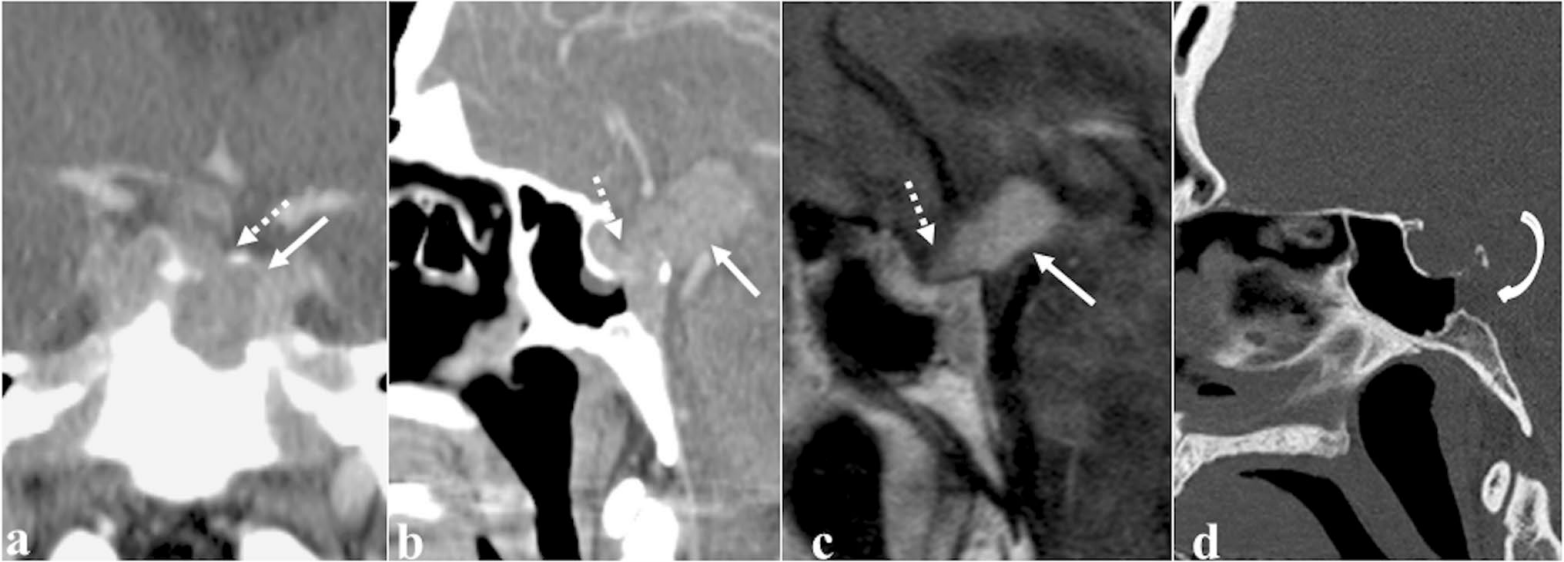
Invasive pituitary macroadenoma in a 60-year-old male patient with visual field defect. A huge pituitary macroadenoma (white arrows) extending into the suprasellar region through the pituitary stalk that invades the sella turcica and clivus. The “snowman” sign (white dotted arrows) is nicely depicted on coronal (a)—sagittal (b) CT sections and sagittal MRI T1W CE image (c) since the soft tumour is indented by the diaphragm sellae. This sign helps in differentiating macroadenomas from pituitary fossa meningiomas. Notice the focal erosion of the dorsal aspect of the clivus on the sagittal bone algorithm reconstruction CT image (d, white curved arrow)
